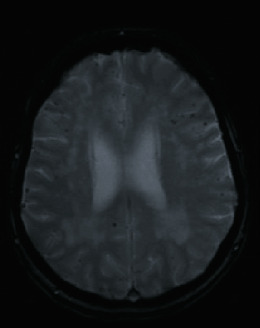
MRI (Gradient echo). 67F, presented with cognitive deficits which were first noticed by close relatives six years prior and initially thought to be due to severe depression. MMSE 18/30, ACER 58/100. MRI demonstrated extensive cerebral amyloid angiopathy.
